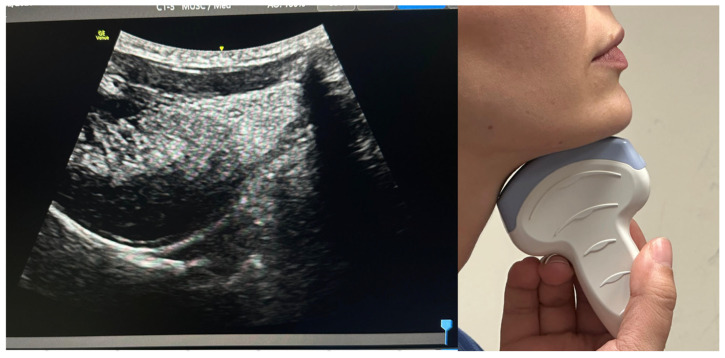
Submental sagittal ultrasound view (curvilinear probe, 3–5 MHz).
